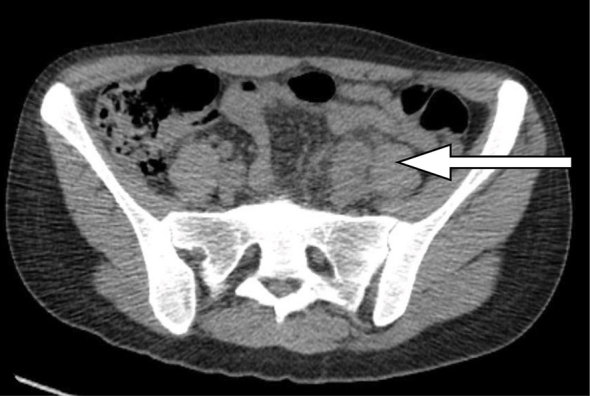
CT kidneys/ureters/bladder performed on admission to the emergency department with left renal colic. The indistinct left iliac vessels and ureter are noteworthy.
